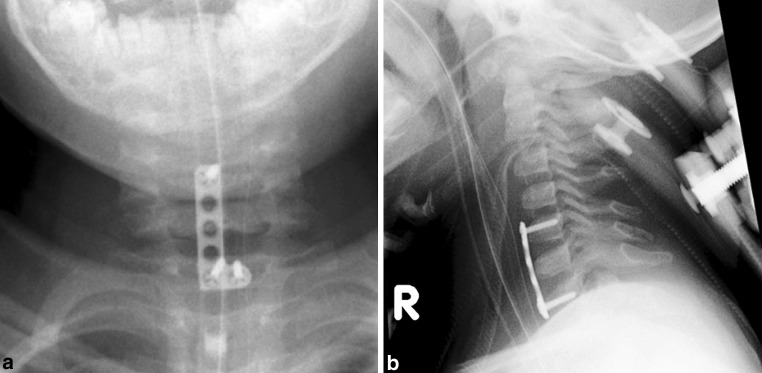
Procedure imaging section. a, b Postoperative radiographs showing the anterior reconstruction of the tension band using a modified T-plate and two screws distally in C7 and one screw proximally in C5. A posterior Ethibond cerclage was performed to augment the injured posterior ligament from C5 to C7
